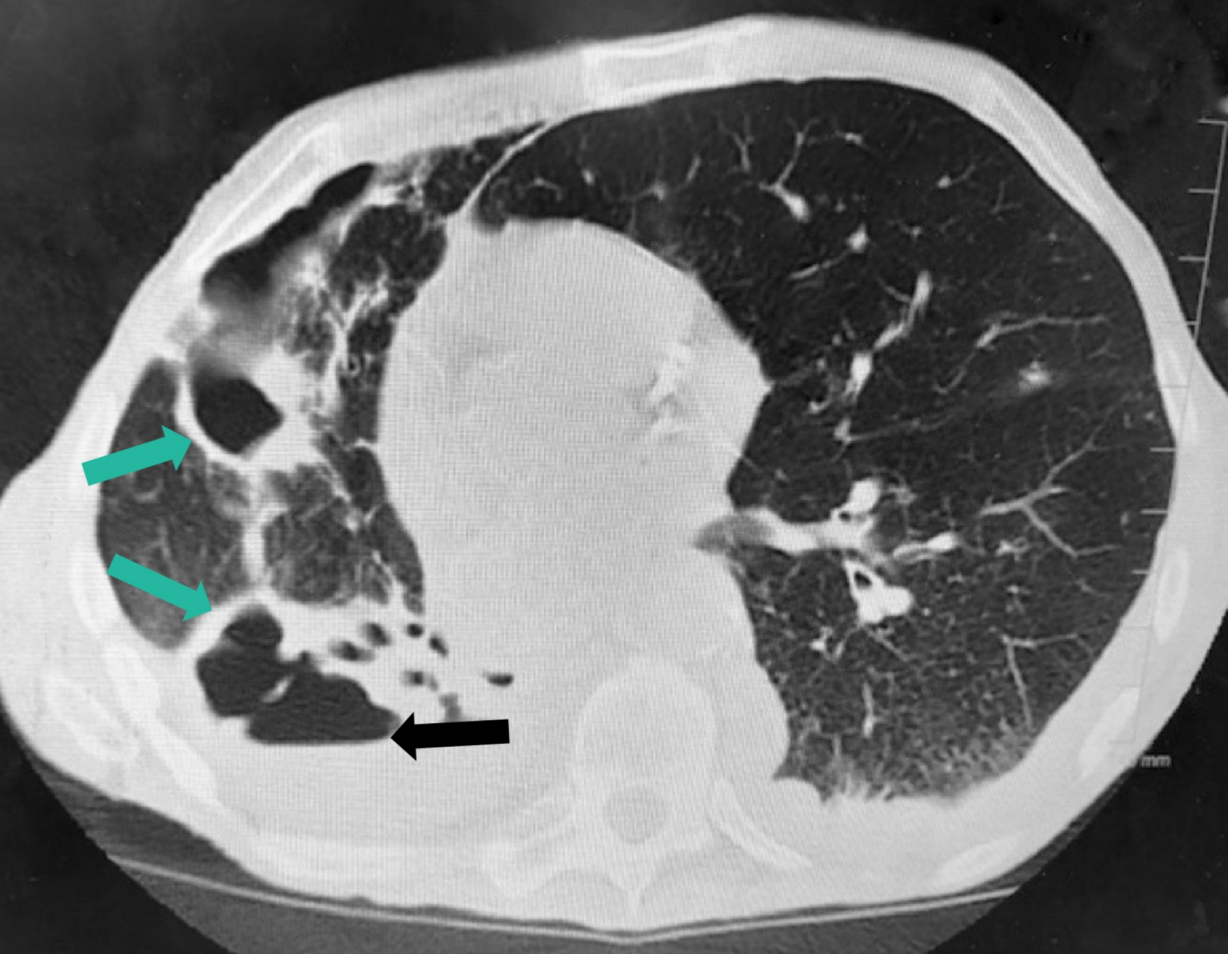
Second CT of the chest without contrast A large thick-walled cavity occupying a majority of the right hemithorax with increase in cavitary lesions (green arrows) in the right middle lobe and overall less aerated lung in the right hemithorax, new areas of parenchymal consolidation, cavitary and noncavitary nodules and patchy ground glass opacities in the left hemithorax with a new small left pleural effusion and a trace right pleural effusion are seen. There is an air-fluid level measuring 6.6 cm x 8.0 cm (black arrow). Findings overall suggest progression of extensive atypical infectious process.
